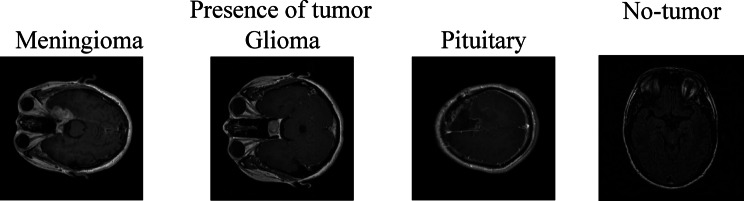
Sample MRI images from the dataset.
